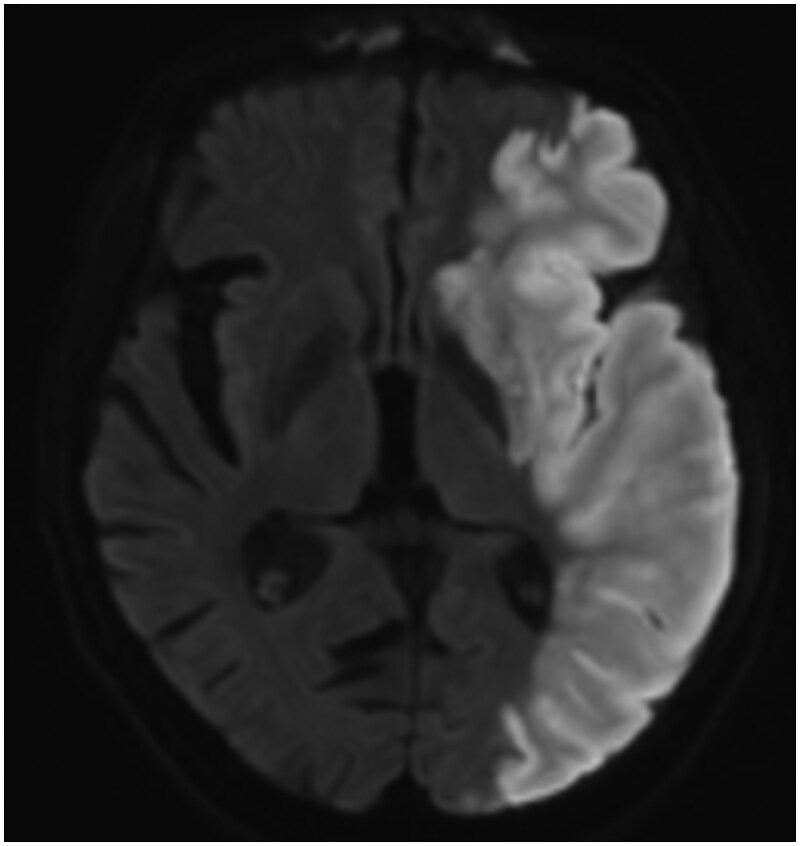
Left MCA territory infarct demonstrated by the DWI signal abnormality.
